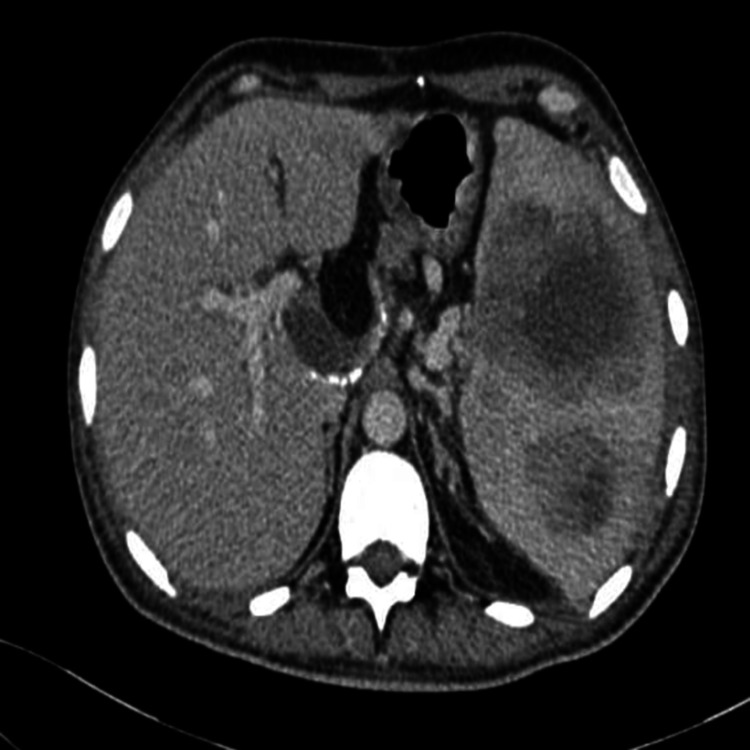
CT axial section showing a splenic abscess CT: computed tomography
